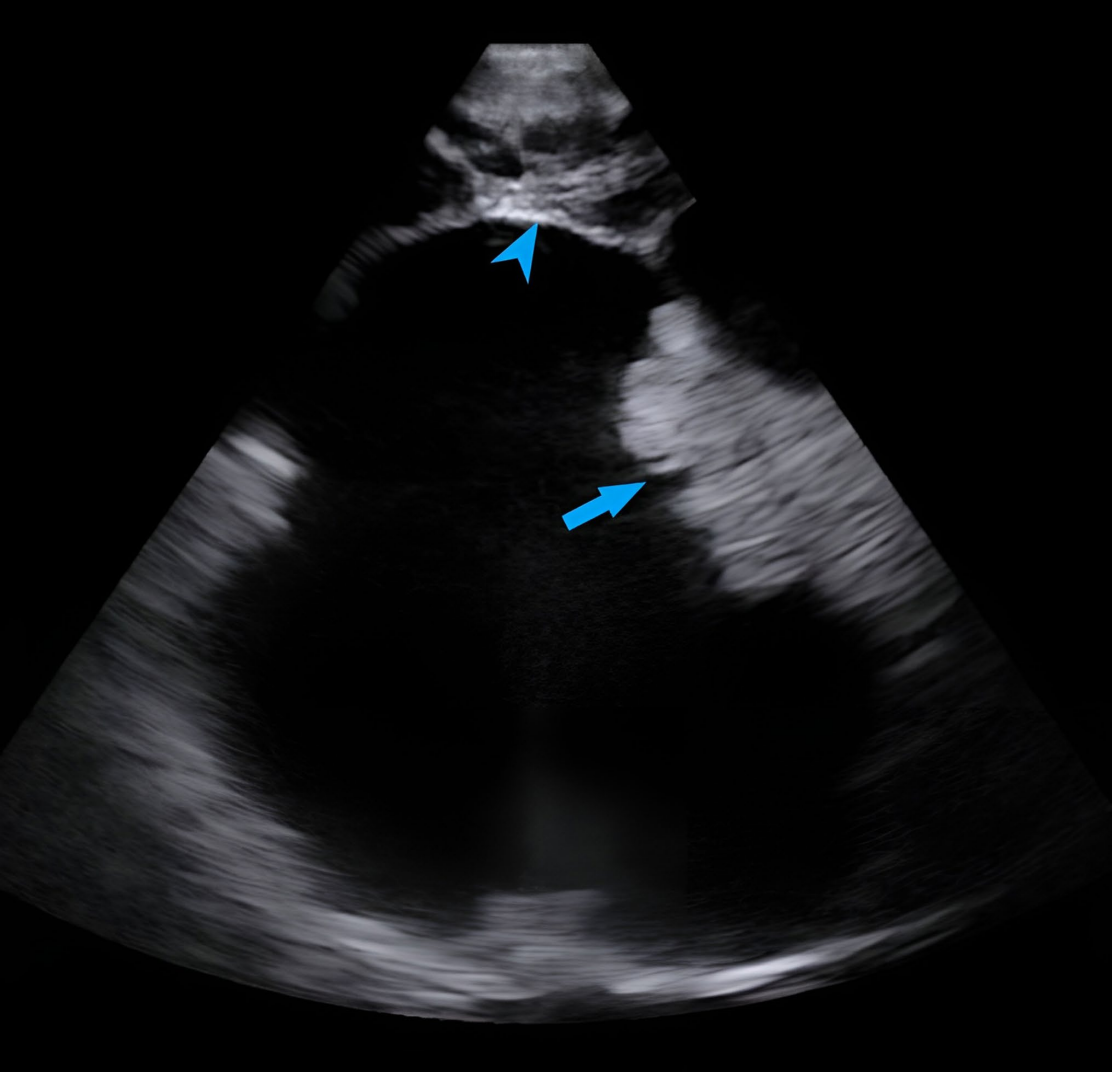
Intraoperative ultrasound images of Case1 (taken after achieving hemostasis and before incising the dura) showing the homogenously hyperechoic solid component with lobulated borders (blue arrow) surrounded by hypoechoic cyst with distinct margins (blue arrowhead)
